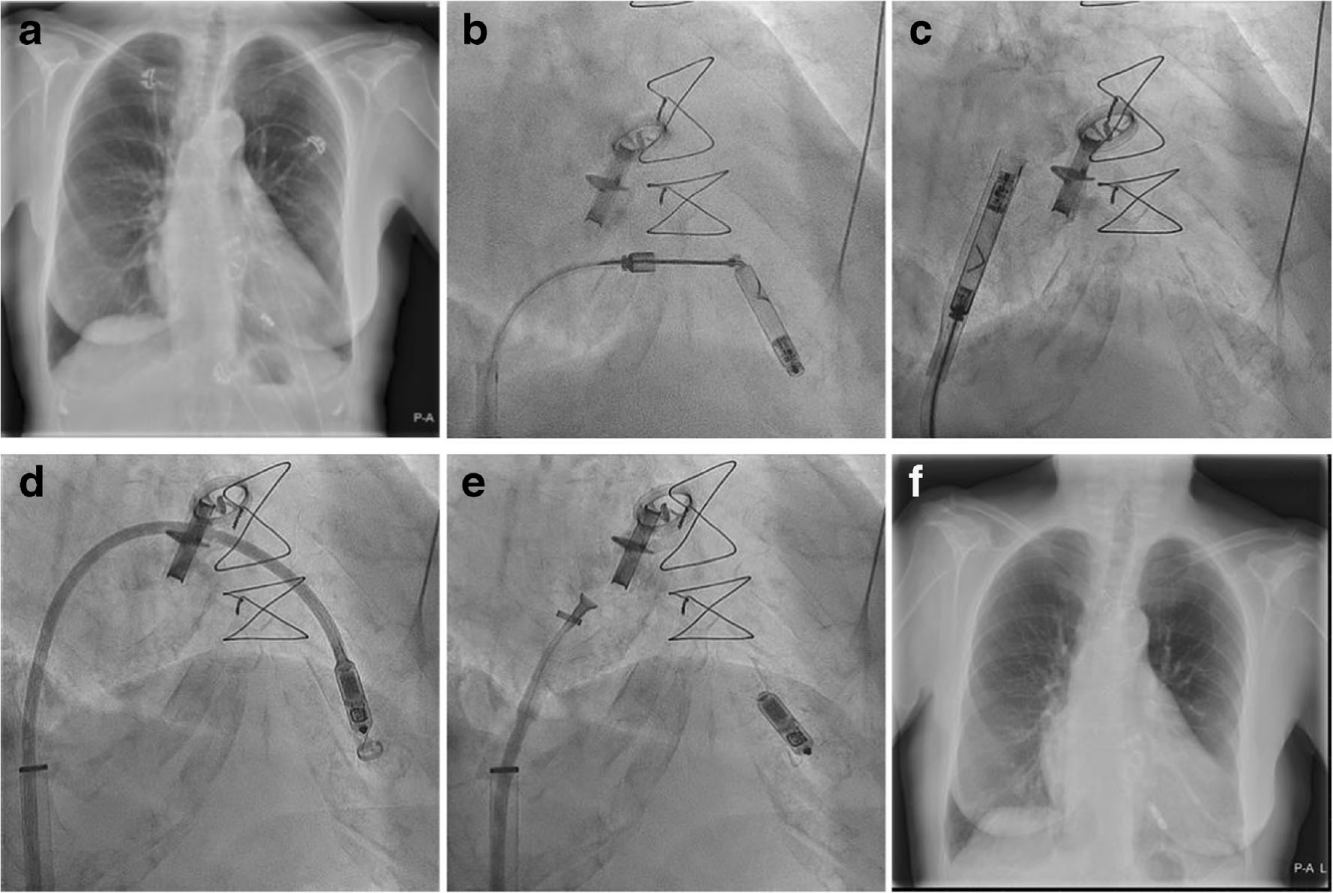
a Chest radiography of the *in situ* Nanostim. b Capture of the distal cap of the device by the snare at the end of the retrieval catheter. c Nanostim removal from the RV. d Micra TPS insertion in the RV. e Micra fixation in the right ventricular myocardium at a slightly different location (i.e., more septal) with the nitinol tines. f Chest radiography of the *in situ* Micra TPS
